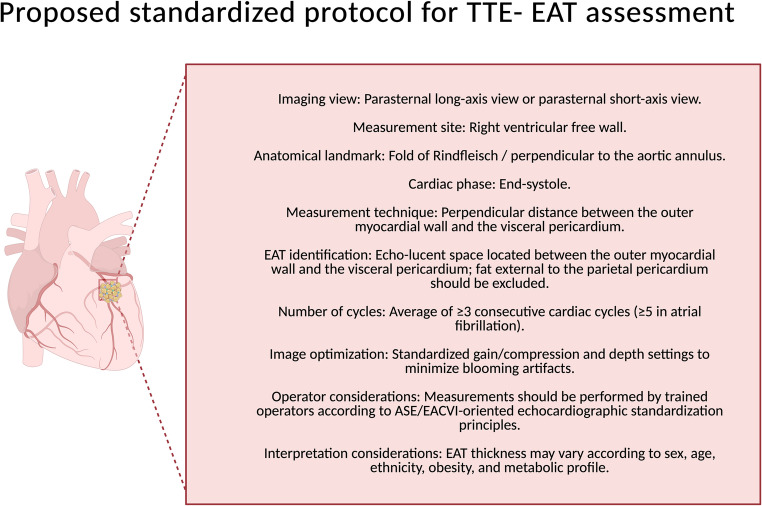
Proposed standardized protocol for transthoracic echocardiographic assessment of epicardial adipose tissue. The figure summarizes a pragmatic stepwise approach for TTE-derived EAT thickness assessment based on the most reproducible methodologies reported in the literature. Recommended acquisition includes measurement at end-systole in the parasternal long-axis view over the right ventricular free wall, using the aortic annulus as an anatomical reference and averaging at least three cardiac cycles. Technical optimization should include appropriate gain and compression settings and careful differentiation between epicardial fat, pericardial fat, and pericardial effusion. The protocol also emphasizes systematic reporting of imaging view, cardiac phase, and measurement methodology in order to reduce operator dependency and improve inter-study comparability. Prospective validation studies are warranted before clinical implementation
